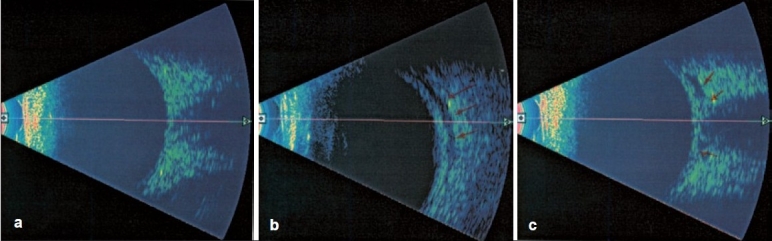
(a) The ultrasound of the eye preinjection with axial scan pass through the optic nerve. (b) The ultrasound 5 minutes after injection of the local anesthetics in the longitudinal axial plan at 6 a.m. of the globe, the red arrows indicate the hypo-echogenic track of the tracked local anesthetics in the subtenon space. (c) The same globe 10 minutes after injection with axial plan through the optic nerve and the red arrows demonstrate the hypo-echogenic pattern around the optic nerve giving the characteristic T sign of perfusion of local anesthetics
